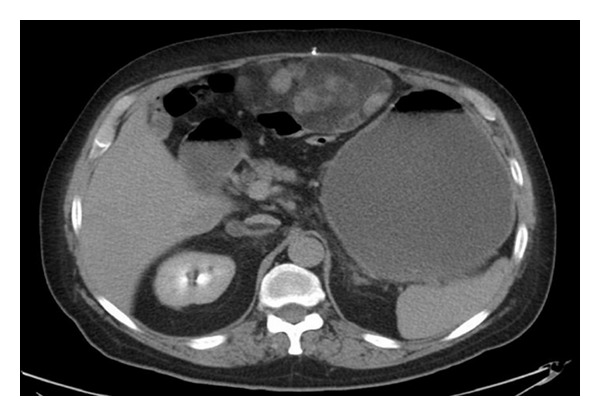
Computed tomographic axial image demonstrating anterior epigastric mass and dilated stomach and duodenum.
